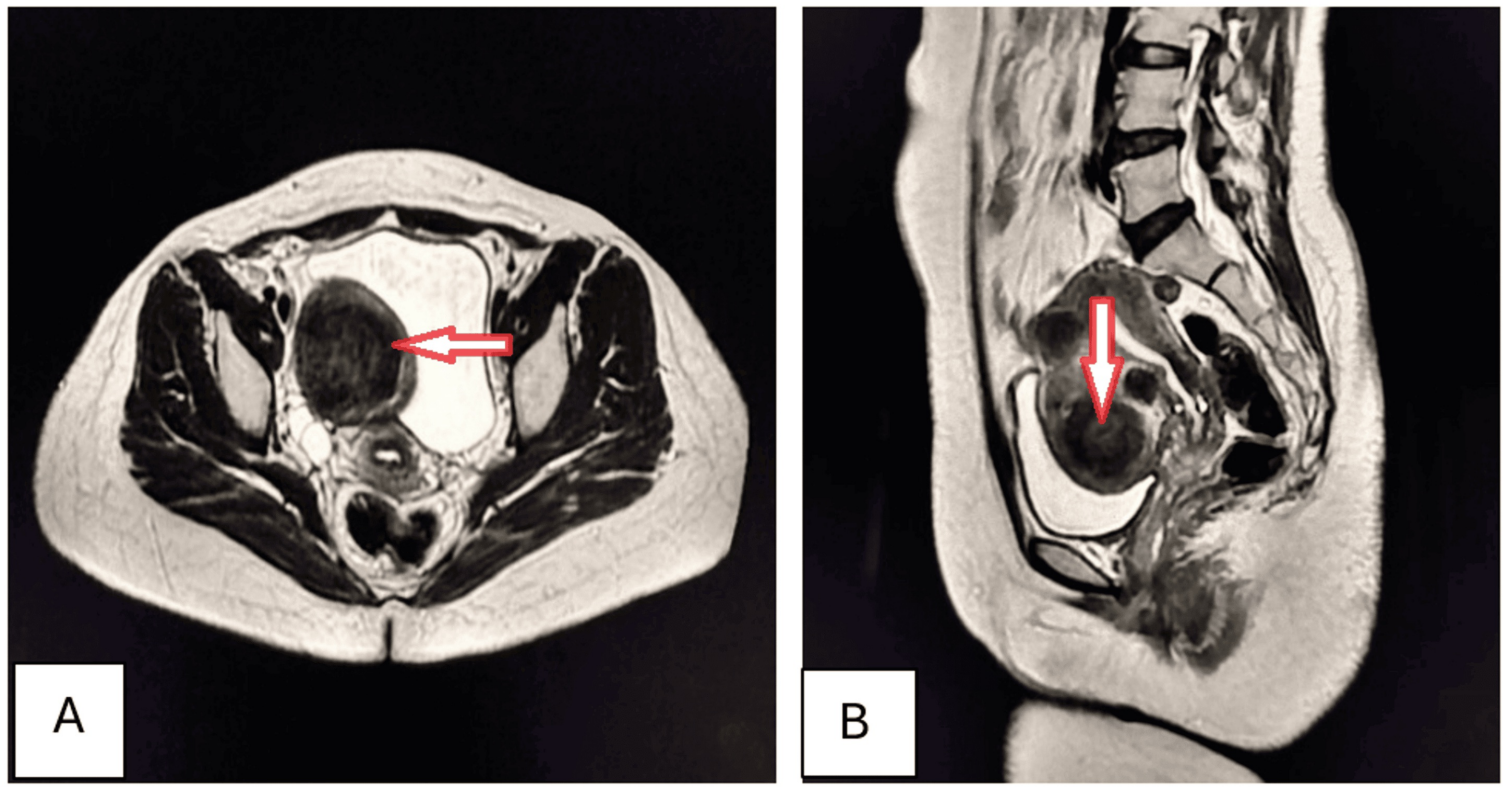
Intramural uterine fibroid (arrows). Axial (A) and sagittal (B) T2W MRI imaging shows a well-defined, round-to-oval, homogenously hypointense mass in the anterior myometrium, consistent with intramural fibroids. It is surrounded by similar, smaller fibroids. Mass effect is noted as indentation over the superior aspect of the bladder and into the endometrial canal. T2W: T2-weighted
